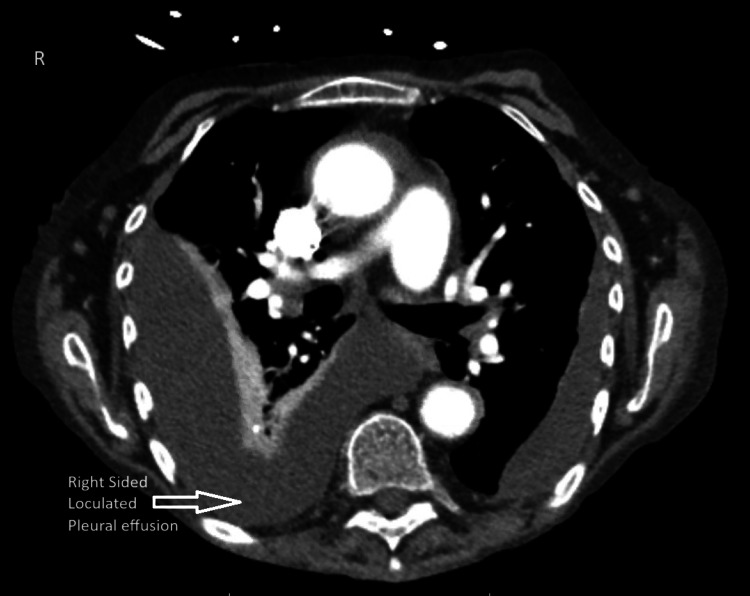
Axial view of CT scan chest showing large right-sided loculated effusion and medium to large left-sided effusion CT: computed tomography
